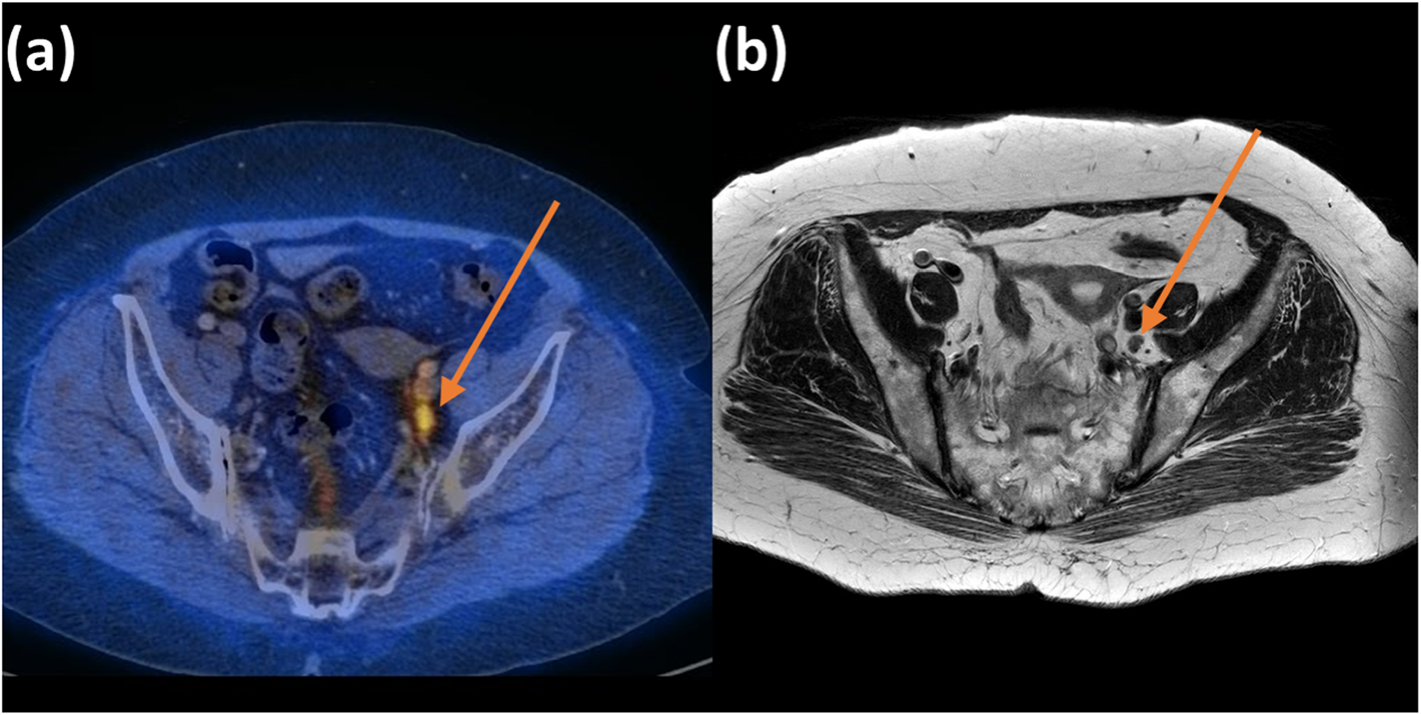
A case of a 69-year-old patient International Federation of Gynaecology and Obstetrics (FIGO) staged IIA with a sub-centimetre pelvic lymph nodes on parametric maps of (a) axial fused ^18^F-fluoro-deoxyglucose positron emission tomography and computed tomography (FDG-PET/CT) image and (b) axial T2-weighted (T2W) image. This patient was classified as metastatic by the IVIM models and correctly staged by the subspecialist but not the non-subspecialist.
